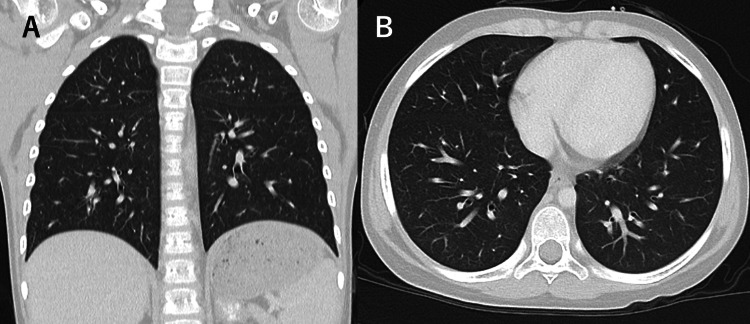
Chest CT showing progression of air trapping six weeks after onset Coronal (A) and axial (B) chest CT obtained six weeks after onset demonstrates progression of air trapping, with diffuse hyperinflation involving almost the whole lung compared with the earlier study CT: computed tomography
